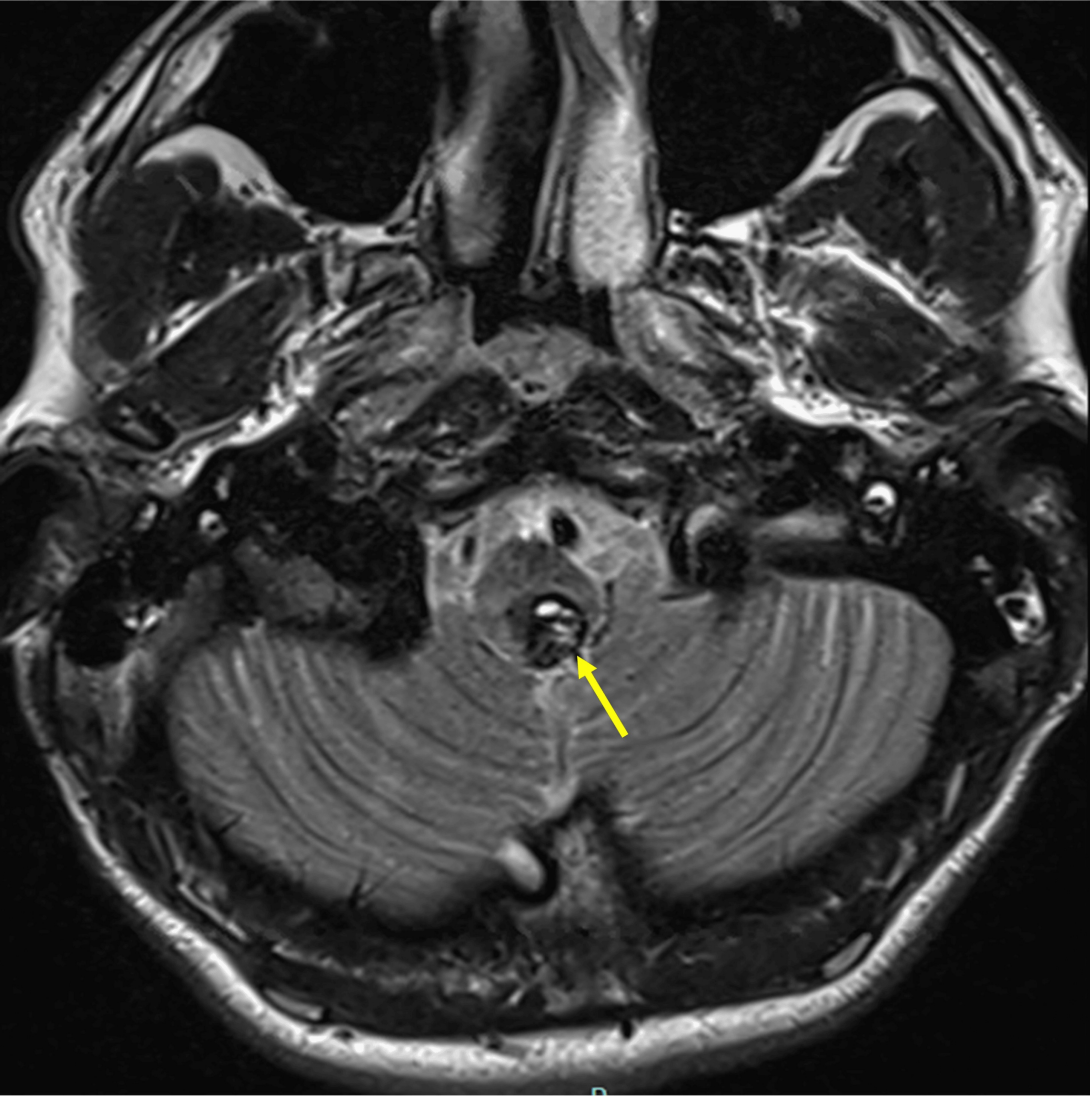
MRI Axial T2 Slice Axial T2-weighted MRI showing a heterogeneous lesion in the dorsal medulla at the level of the area postrema (arrow), with mixed signal intensity and surrounding edema. MRI: Magnetic resonance imaging
